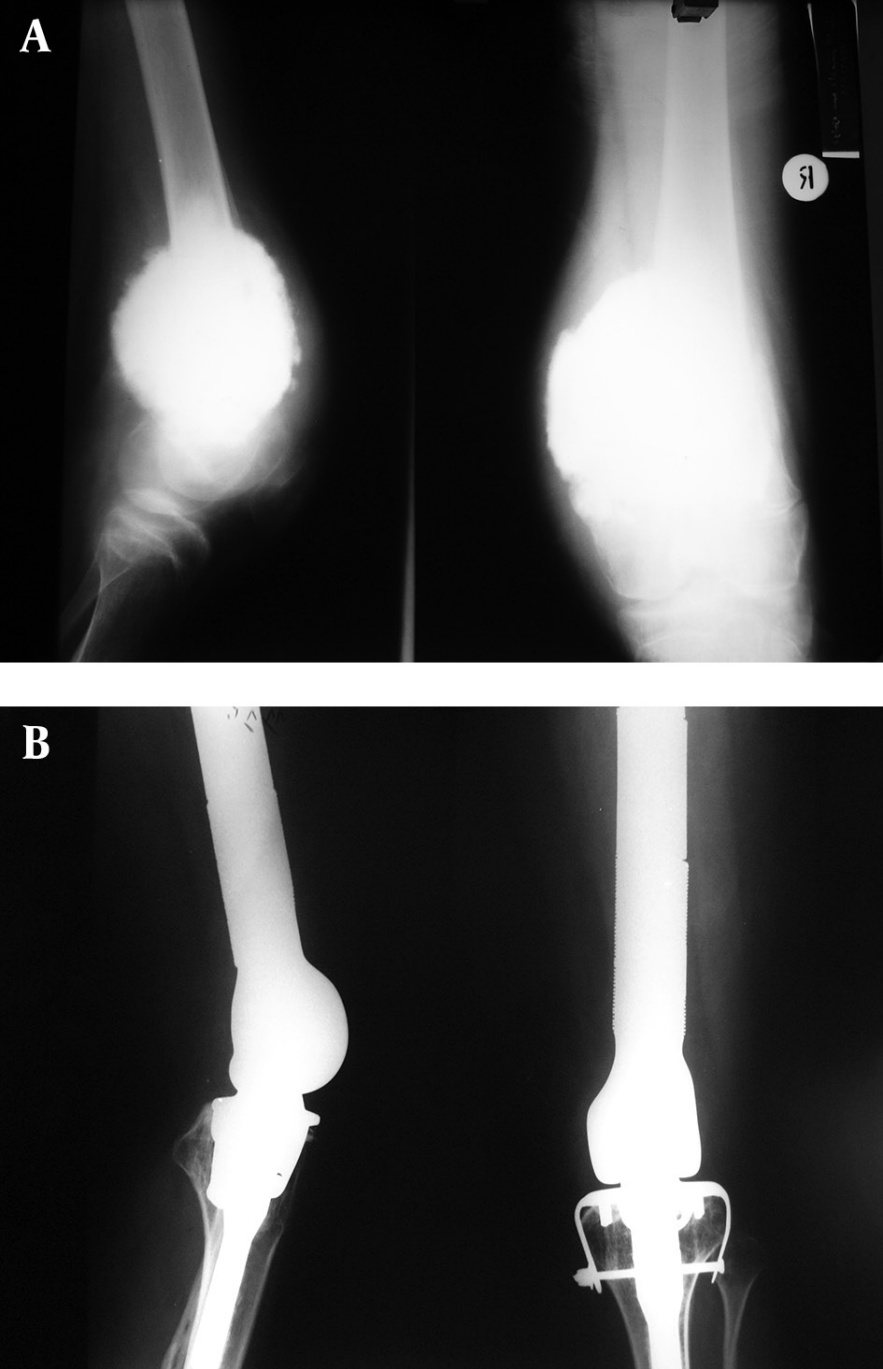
Anteroposterior and Lateral X-rays of the Right Knee Showing Distal Femur Conventional Osteosarcoma (A). Post Total Knee Arthroplasty X-rays After Knee Fusion (B).
